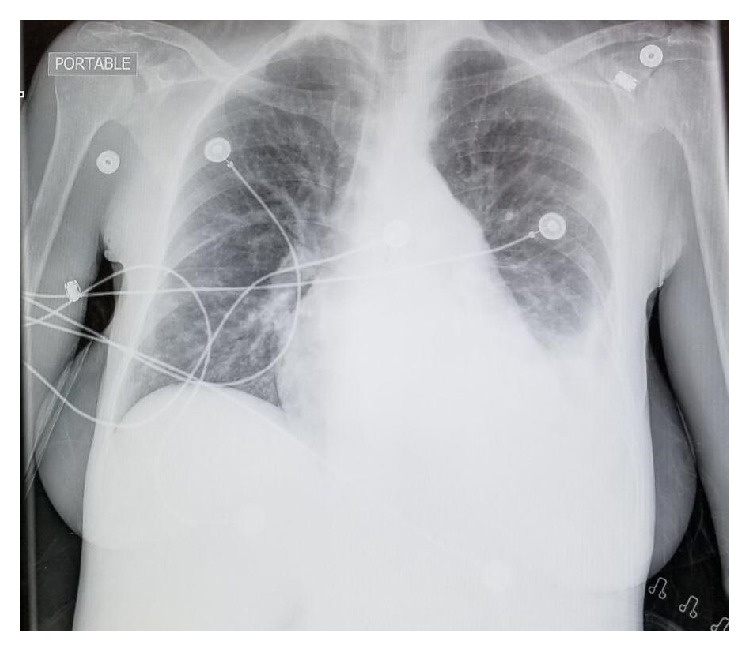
Initial chest X-ray demonstrating an isolated left-sided pleural effusion.
